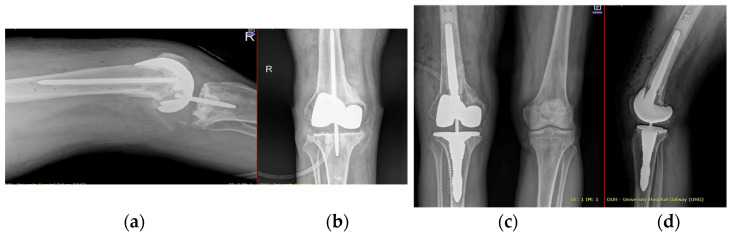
Radiographic findings demonstrating customized spacer and final implants at final follow-up of case 1. (a,d) Lateral view. (b,c) Posterior view. (d) Anterior view.
